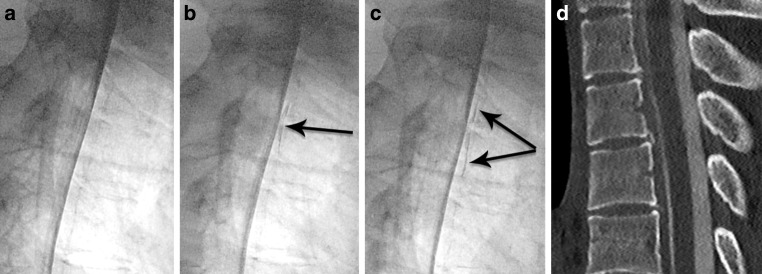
Middle-aged female with sudden onset of orthostatic headache after a mild trauma. Dynamic myelography in prone position with progressive tilt of the table with time course from a–c, total time: 10 s. a Before and b immediately after Valsalva maneuver (*black arrow* showing the precise location of the leak) with increasing leakage of contrast medium (*black arrows*) into the ventral epidural space c. d Postmyelography CT demonstrating a prominent ventral microspur at the corresponding level
